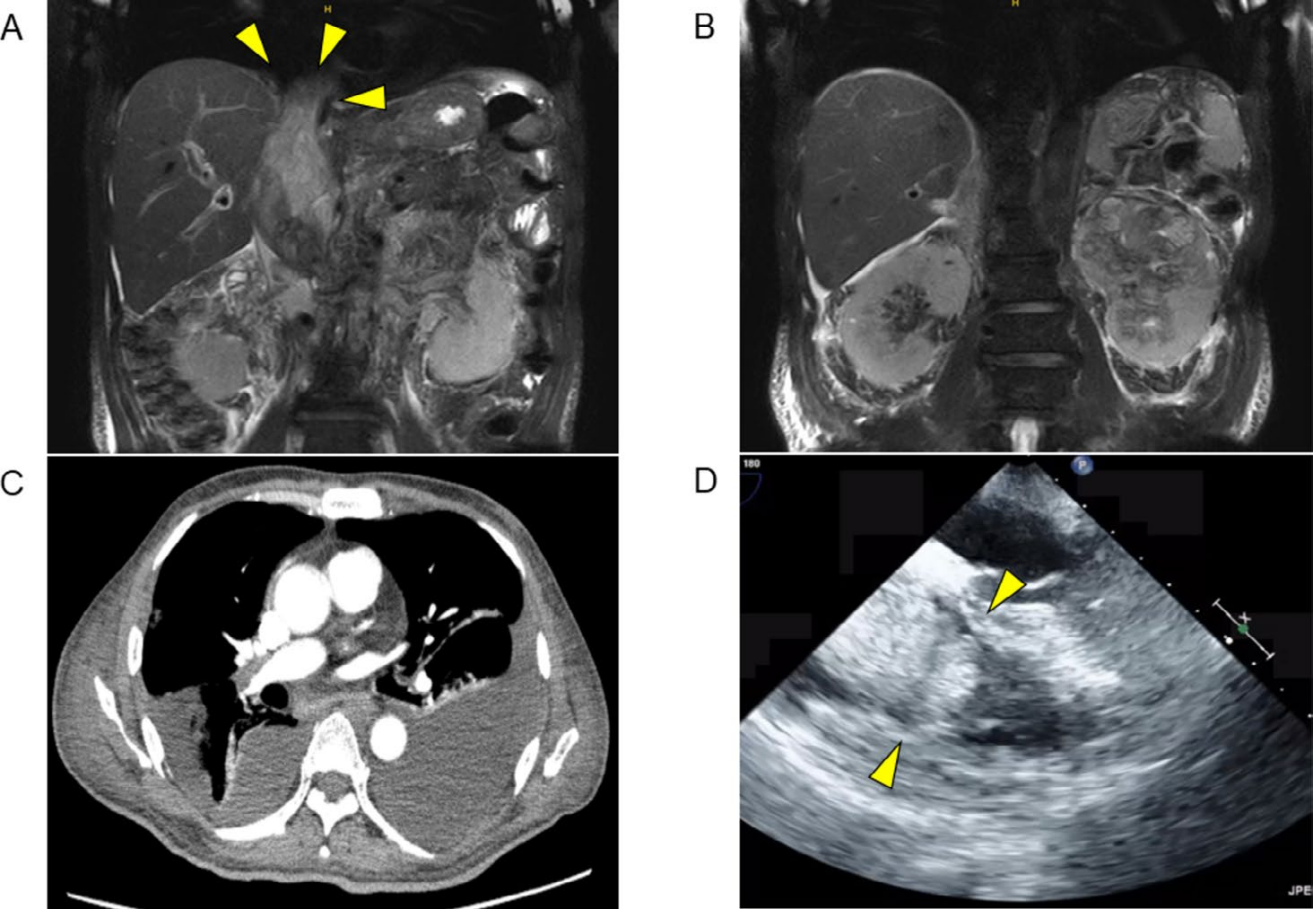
(A, B) T2‐weighted MRI (coronal view). (A) Tumor thrombus in the IVC (arrowhead indicates the cranial end of the IVC tumor). (B) Primary renal tumor. (C) Pulmonary embolism in the right pulmonary artery. (D) Transthoracic echocardiography (arrowhead indicates the tricuspid valve, with the tumor thrombus moving in synchrony with cardiac motion).
